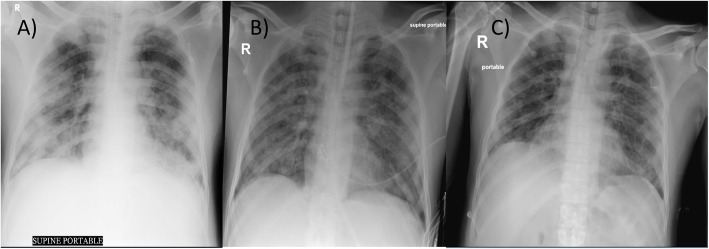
Case 70: 47-year-old male, overweight (BMI 27.88) stayed at the ICU for 14 days, score 7, initially intubated, with post-critical polyneuropathy and grown Staphylococcus epidermidis Isolated from blood culture, resistant to Benzylpenicillin, Clindamycin, Erythromycin, Fusidic Acid, Oxacillin, Trimethoprim/Sulfa. Chest X-ray: a day of admission (April 17th). Extensive areas of diffusely organized consolidation throughout both lungs in all lobes, almost involving most of the lower lobes. b Day 4 after treatment (April 21st). X-ray partial resolution of the disease noted. c Day 13 after treatment (April 30th). Bilateral patchy opacities with mid to lower lung zone predominance as compared with previous partial resolution of the disease noted
